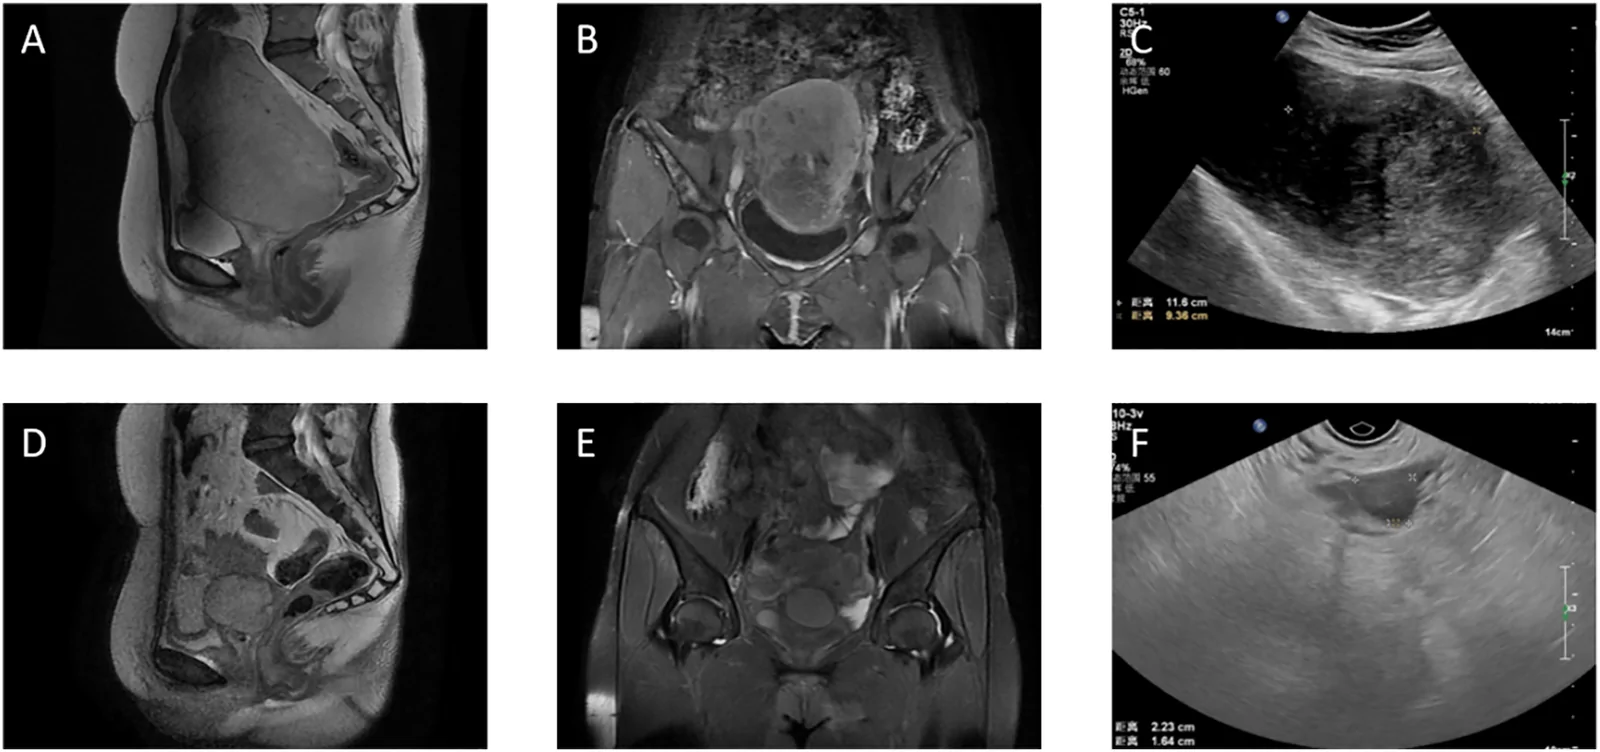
Comparison of pelvic MRI and gynecological ultrasound before and after neoadjuvant chemotherapy in Patient B. (A, B) Pre-neoadjuvant chemotherapy pelvic MRI showed a mass-like lesion in the uterine body and cervix with isointense T1 and hyperintense T2 signals; DWI demonstrated high signal intensity, with a maximum cross-sectional area of approximately 83 mm × 107 mm × 130 mm; (C) Pre-neoadjuvant chemotherapy gynecological ultrasound showed a 11.6 × 9.4 × 9.4 cm hypoechoic mass in the lower uterine segment and cervix, with indistinct borders and an irregular shape; CDFI: relatively rich intravascular blood flow signal; RI: 0.60; (D, E) Pelvic MRI after neoadjuvant chemotherapy showed a mass-like lesion at the cervix with T1-equivalent and T2-high signal intensity; DWI demonstrated slightly high signal intensity; maximum cross-sectional dimensions were approximately 48 mm × 42 mm × 44 mm; (F) Post-neoadjuvant chemotherapy gynecological ultrasound shows a hypoechoic mass in the lower uterine segment and anterior cervical lip, measuring 5.0 × 4.5 × 3.4 cm, with relatively well-defined borders and an irregular shape; CDFI: punctate and linear blood flow signals are observed peripherally.
